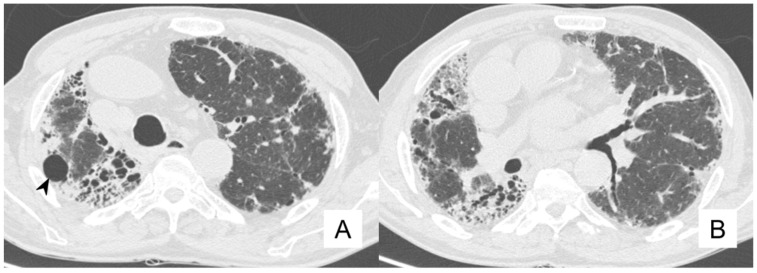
Same patient as the previous figure (Figure 12)—follow-up HRCT performed one year later. Images demonstrate disease progression, with an increased representation of honeycomb and traction bronchiectasis (A,B). These findings were associated with the development of cystic cavities, namely in the right upper lobe (black arrowhead in A).
